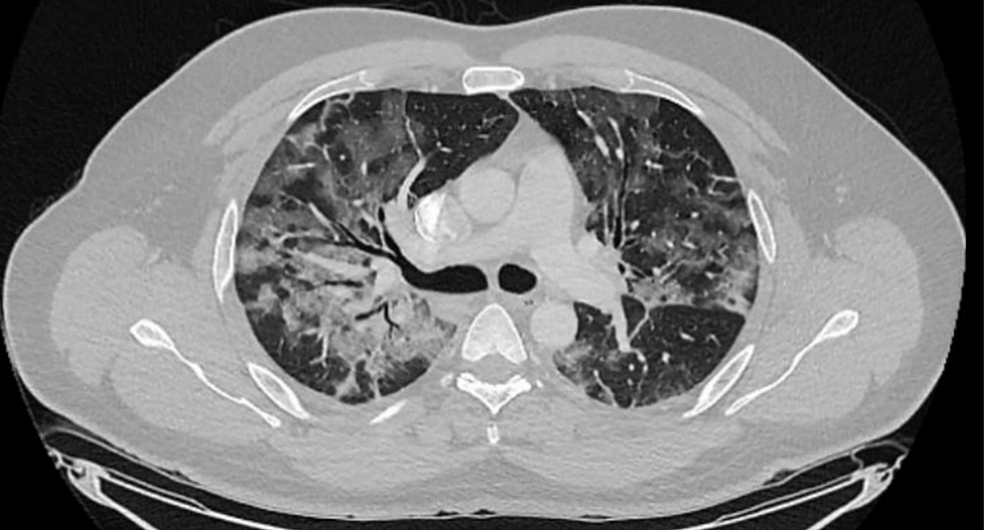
Coronal computed tomography of the chest.
